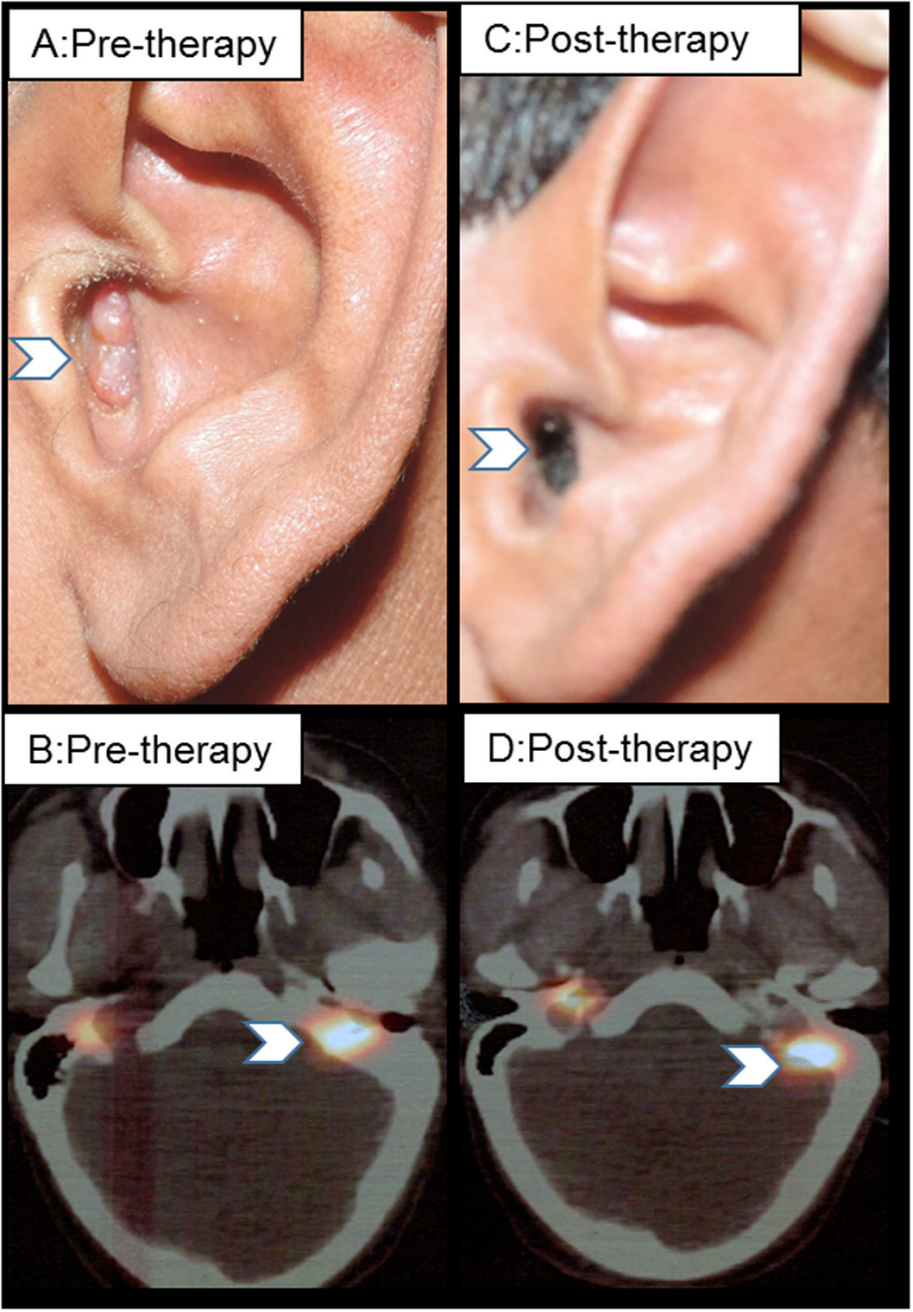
Response in SDH-D positive (case 4) unresectable HNPGL following six cycles of PRRT therapy ((A, B) pretherapy mean SUV_max_: 104 and (C, D) posttherapy mean SUV_max_: 24, respectively).
